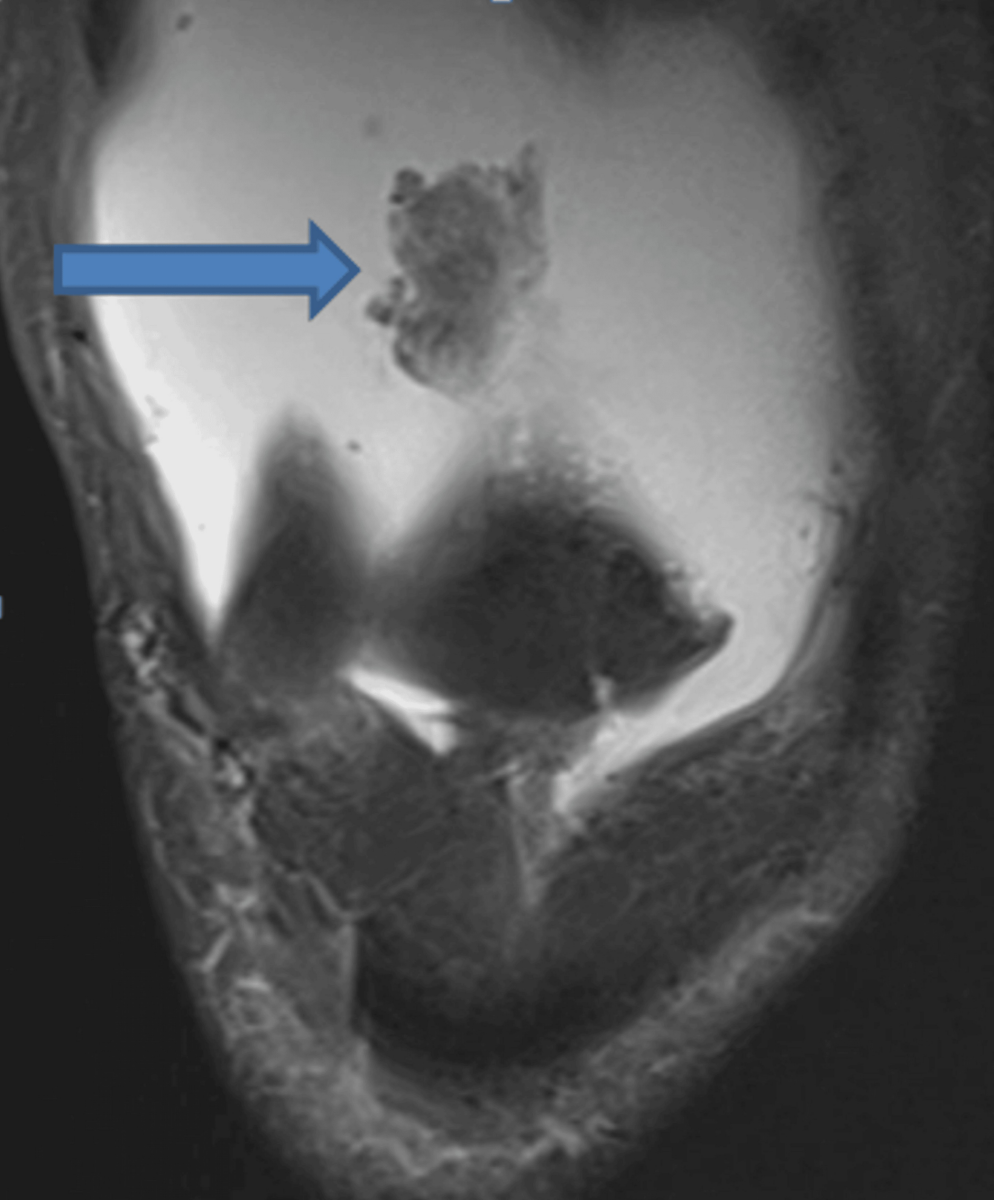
Coronal section of right knee MRI demonstrating leaf-like projections of tissue arising from the suprapatellar pouch, which has the same signal intensity as fat.
